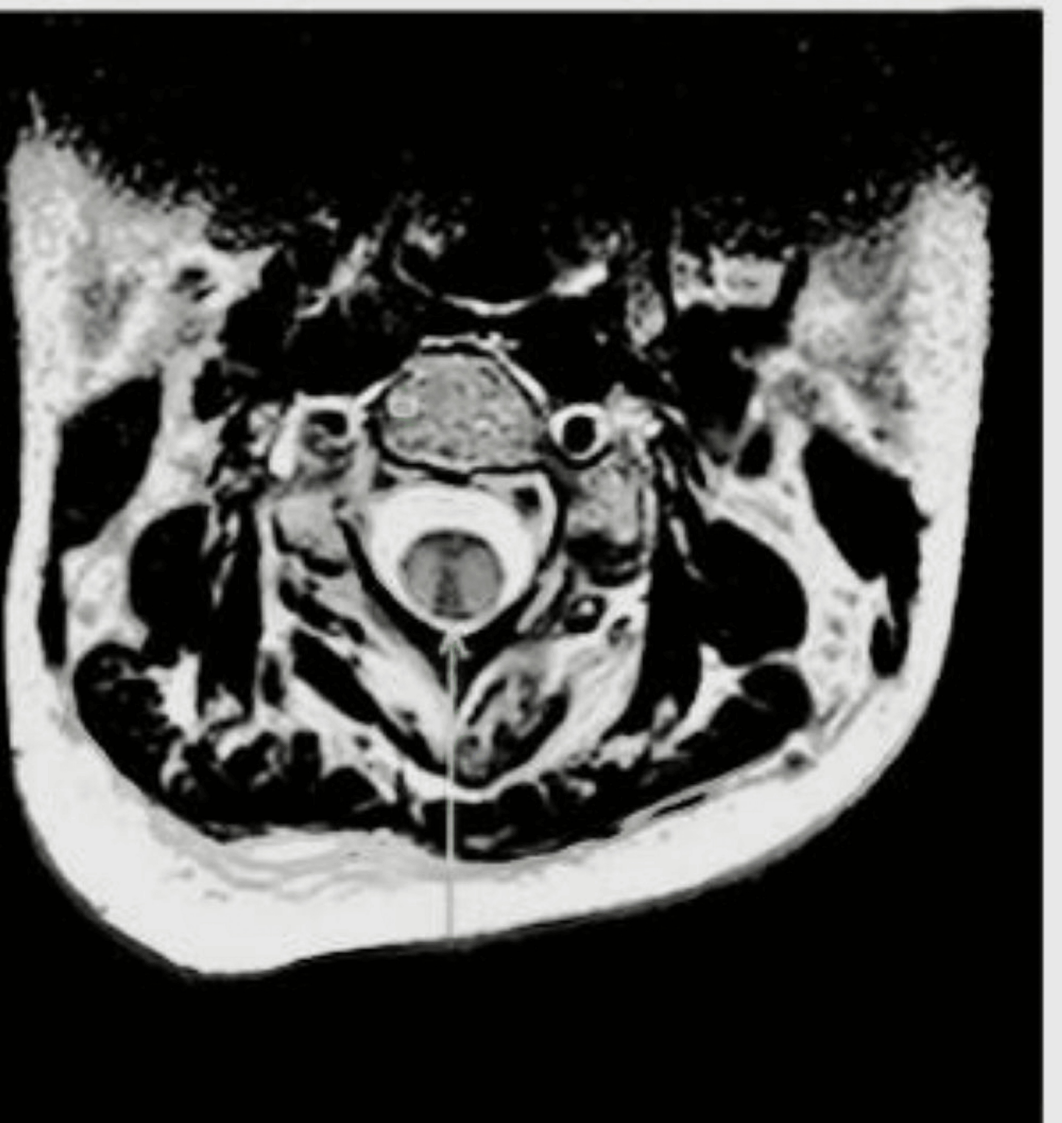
MRI spine (T2-weighted axial section) demonstrates the hyperintensity in the cervical spine Arrow highlights the hyperintensity
